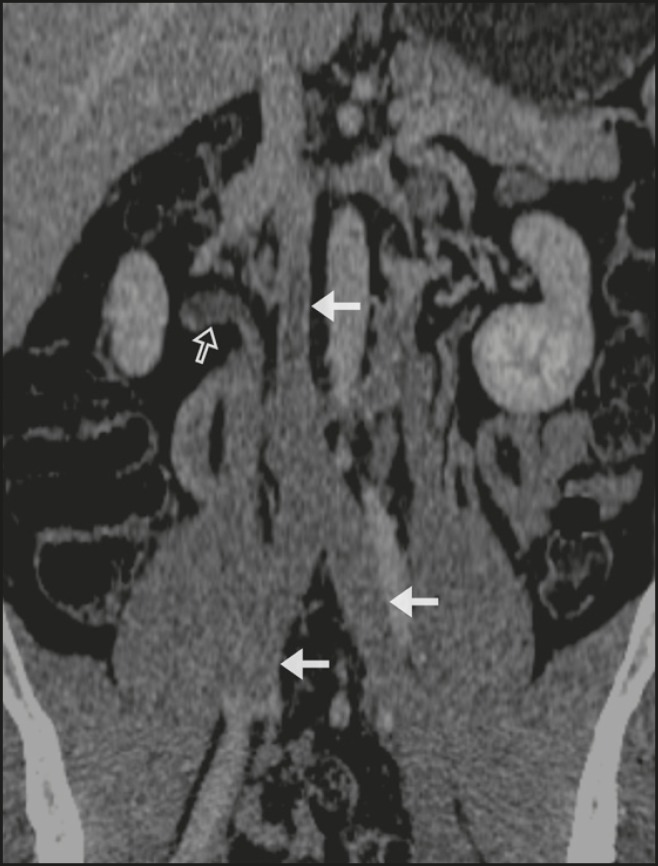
Abdominal coronal reformatted computed tomography scan showing extensive
